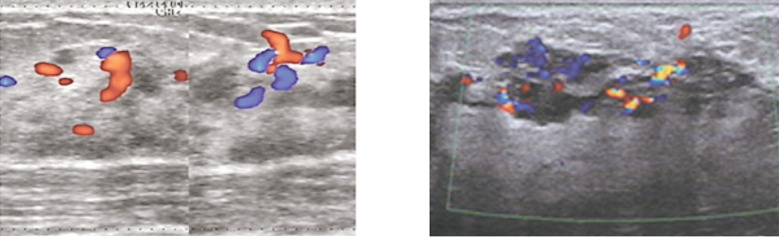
Typical ultrasound blood flows Left: early-stage infiltrating breast cancer, ALN-negative, 30-year-old, with a disease course of 3 months, grade-2 flow signals primarily distributed in the tissue surrounding the tumor and the intralesional streak-like hyperechoic area. Right: mid- to late-stage infiltrating breast cancer, ALN-positive, 36-year-old, with a disease course of 23 months, grade-3 flow signals mainly distributed in the intralesional solid hypoechoic area.
